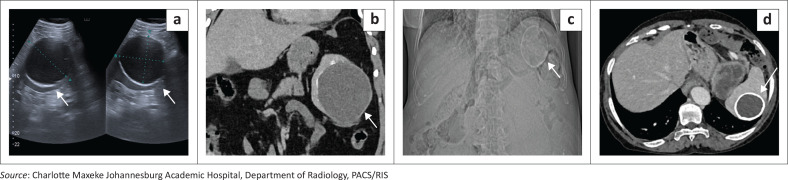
(a, b) Benign non-parasitic splenic cyst in a patient evaluated for a left upper quadrant mass. (a) Ultrasound demonstrates a well-circumscribed hypoechoic cystic lesion without internal septations or mural nodularity, with a thin rim of peripheral calcification (arrows). (b) Coronal contrast-enhanced CT in the same patient confirms a rounded, homogeneous fluid-attenuation cyst within the splenic parenchyma, characterised by a thin discontinuous curvilinear calcified rim (arrow). No internal septations, mural nodules, enhancing solid components or perisplenic inflammatory change are identified. (c, d) Benign non-parasitic splenic cyst identified incidentally in a 67-year-old male undergoing evaluation for renal calculi. (c) Scanogram (AP radiograph) demonstrates a well-circumscribed rounded lesion in the region of the spleen with a conspicuous peripheral calcified rim (arrow). (d) Axial contrast-enhanced CT confirms a cystic splenic lesion with a thick continuous calcified wall and homogeneous fluid attenuation, without internal septations, mural nodules or enhancing solid components (arrow).
